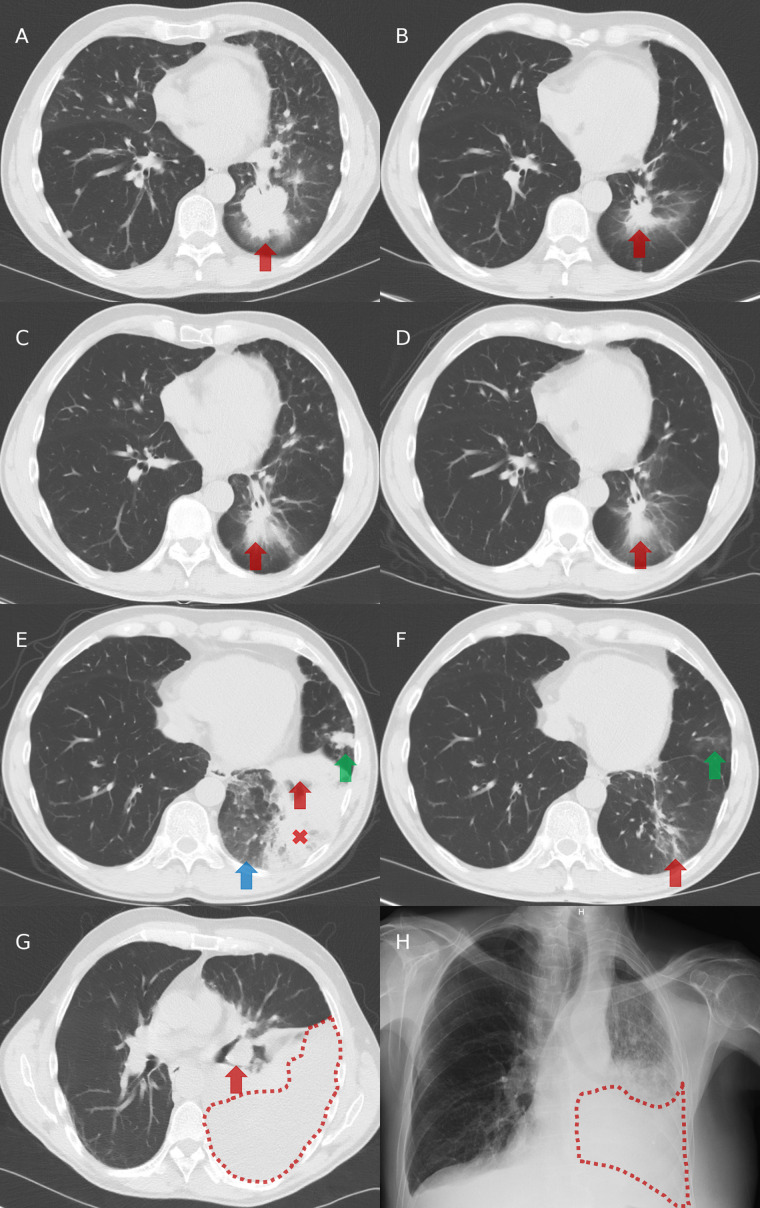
Chest imaging evolution. (A) Computed tomography (CT) at diagnosis: presence of a left-lower lobe primary tumor (red arrow). (B) CT after three cycles of cisplatin and pemetrexed in first-line: partial tumor response with decreased size of the primary tumor (red arrow). (C) CT after 7 cycles of pemetrexed in maintenance and then 2.5 months without systemic treatment: tumor progression with increased size of the primary tumor (red arrow). (D) CT after two months on crizotinib in second-line: partial tumor response with decreased size of the primary tumor (red arrow). (E) CT after 5.5 months on crizotinib in second-line: progression of the primary tumor (red arrow), appearance of ground glass opacities (blue arrow), a retro-obstructive condensation in the left lower lobe (red cross), and a nodular lesion in the lingula (green arrow). (F) CT after two months on alectinib in third-line: partial tumor response with decreased size of the left lower lobe primary tumor (red arrow) and of the lingular nodule (green arrow). (G) CT after 15 months on alectinib: tumor progression with increased size of the primary tumor (red arrow) and appearance of a left pleural effusion (area under red dots). (H) Radiography after two months on BRAF/MEK inhibitors in fourth-line: left pleural effusion (area under red dots).
